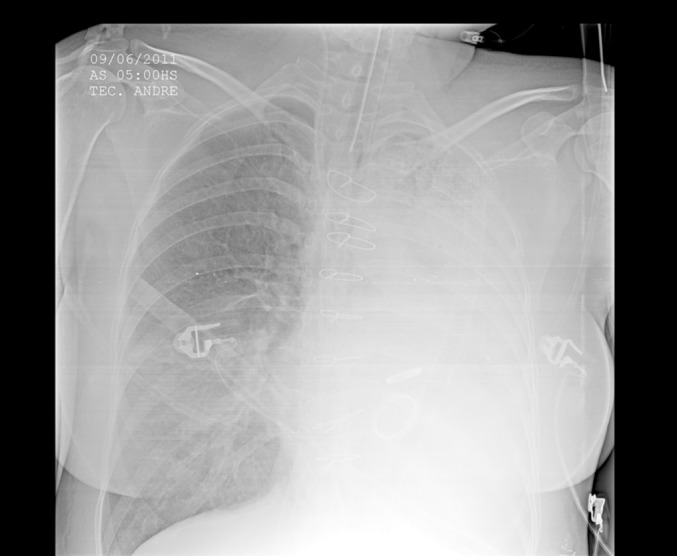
Chest X-ray at admission revealing extensive opacity in the entire left
